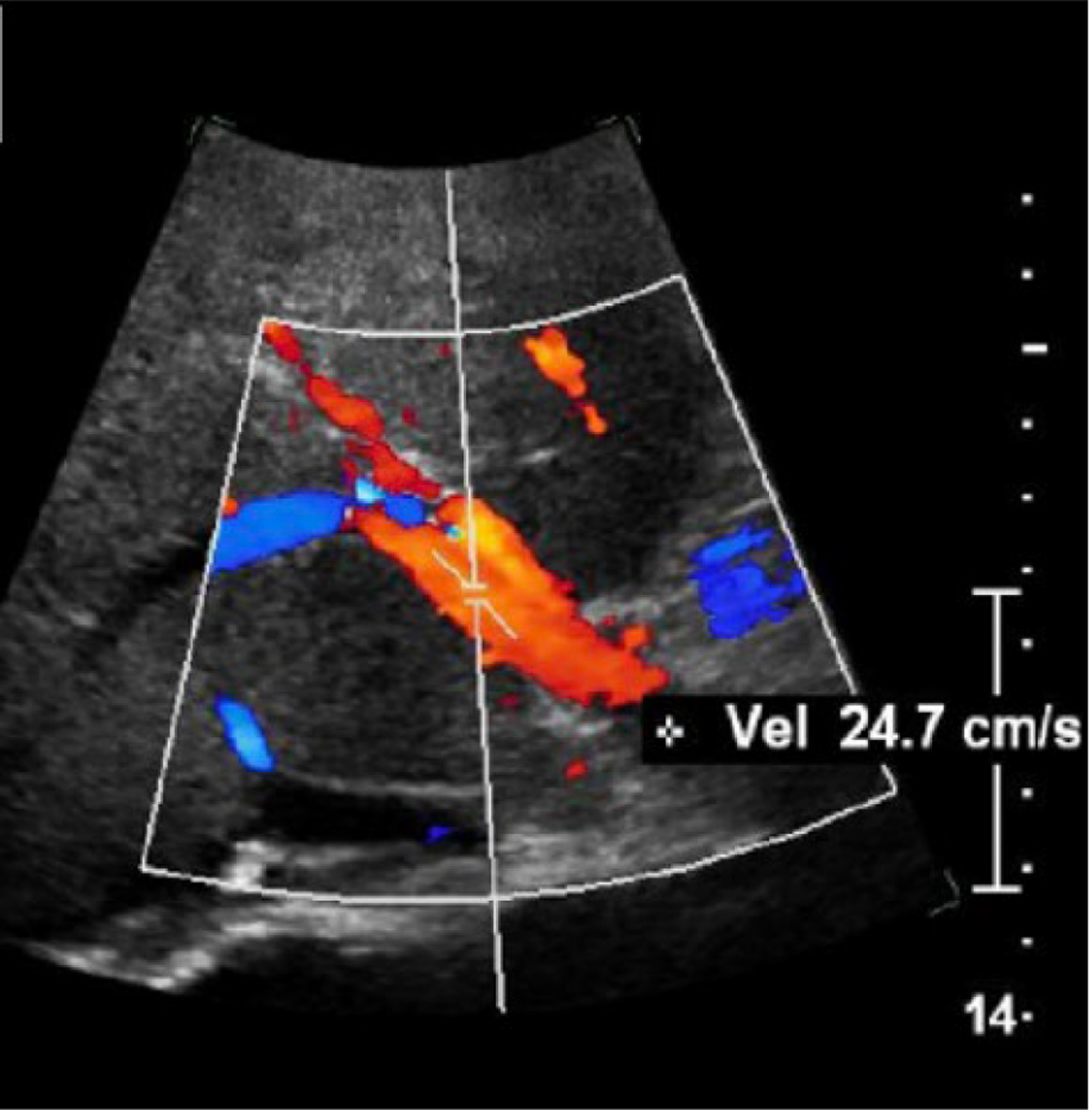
Doppler study of portal vein showed complete recanalization with normal blood flow.
